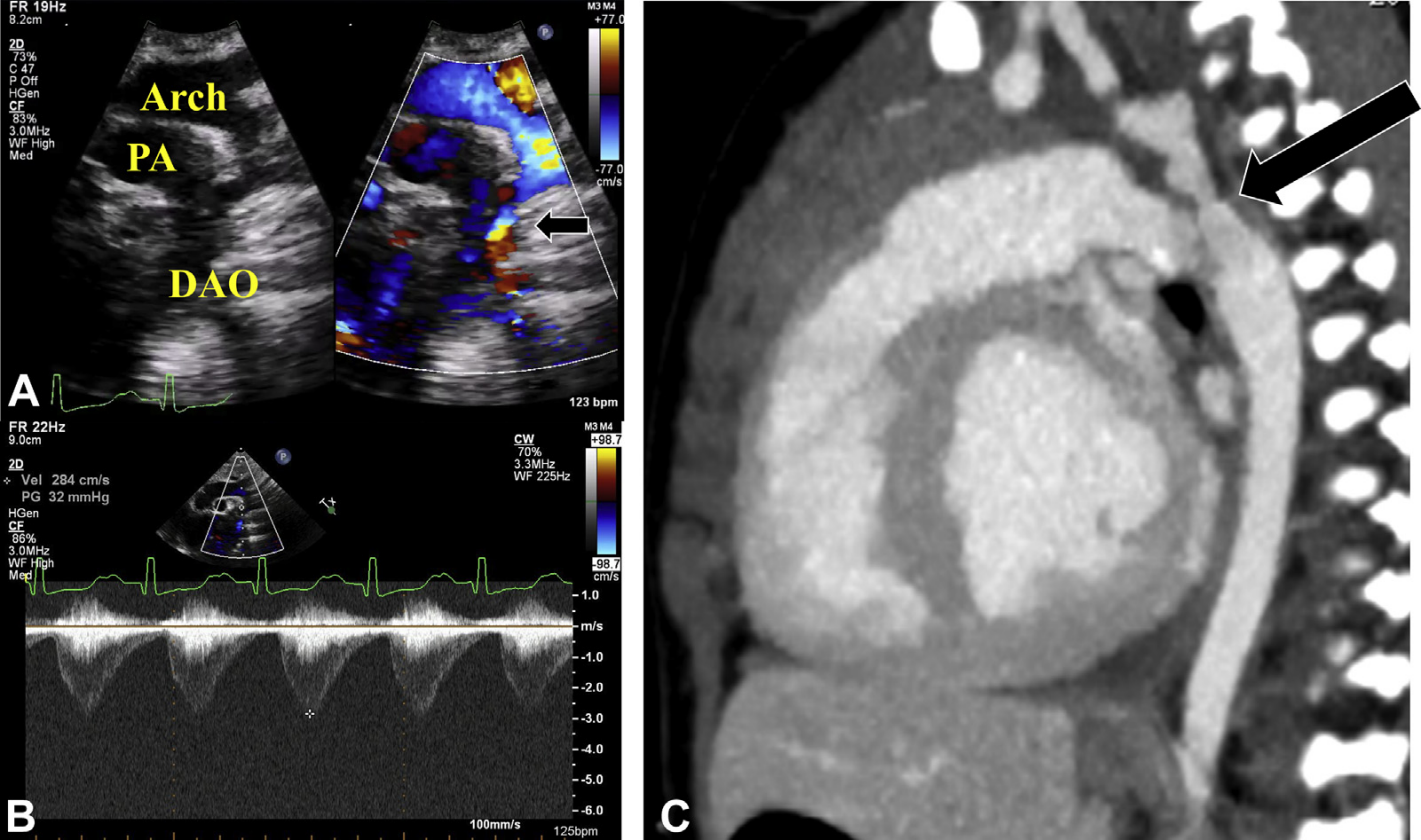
(A) Transthoracic long-axis aortic arch view from the suprasternal notch window showing flow acceleration across aortic isthmus (*black arrow*) and (B) increased peak velocity (2.8 m/sec with a calculated peak gradient of 32 mm Hg) by continuous-wave Doppler assessment. (C) Sagittal view on cardiac computed tomography showing a narrowed aortic isthmus (*black arrow*). *DAO*, Descending aorta; *PA*, pulmonary artery.
